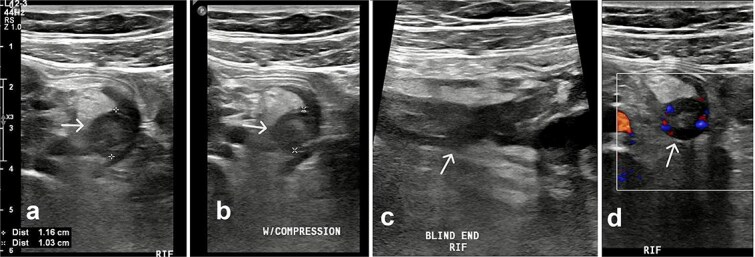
Ultrasound of the right iliac fossa. Transversal ultrasound image of the appendix (white arrow). (a) Before compression. (b) During compression, showing an uncompressible distended appendix (cursors). (c) At the level of the right iliac fossa, there is a blind-ending tubular structure.
